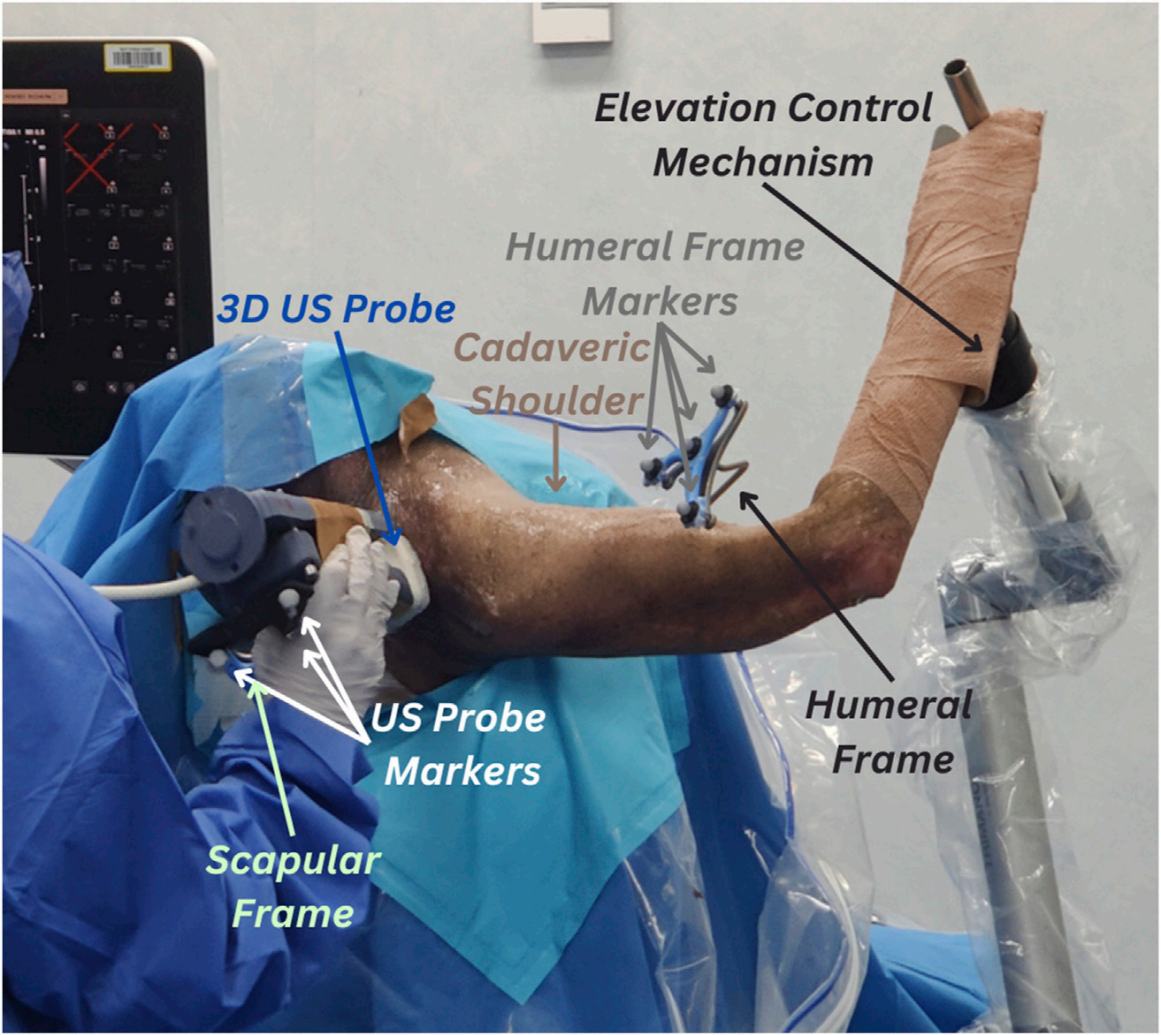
Experimental setup for 3D ultrasound (US) tracking of the shoulder bones. Cadaveric shoulder is secured in a beach chair setup and arm elevation is set to high using the elevation control mechanism. The protocol uses retroreflective marker clusters tracked via the VICON motion capture system. The first cluster is attached to the humeral reference frames, the second cluster (partly concealed in the figure) is attached to the scapular frame and the third cluster is attached to the 3D US probe.
